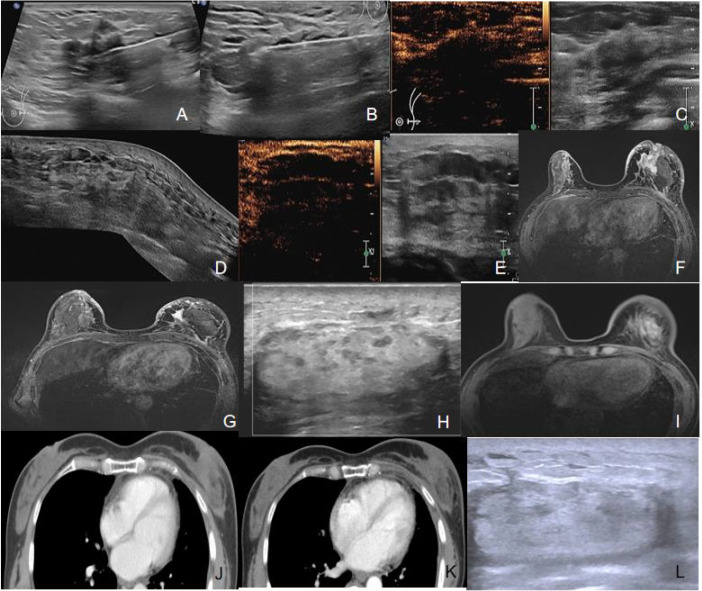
Long-term imaging follow-up after tumor ablation. (A) Pre-ablation biopsy; (B) Intraoperative ablation under ultrasound guidance; (C) Immediate post-ablation contrast-enhanced ultrasound showing no enhancement in the target area; (D) 1-month post-ablation panoramic ultrasound of ablation zone; (E) 1-month post-ablation contrast-enhanced ultrasound demonstrating no enhancement; (F) 1-month post-ablation enhanced MRI showing lesion size reduction compared to baseline; (G) 6-month post-ablation enhanced MRI indicating volume reduction in majority of lesions; (H) 6-month post-ablation ultrasound revealing no discernible mass echo in the region; (I) 1-year post-ablation enhanced MRI demonstrating significant regression of the lesion; (J) 2-year post-ablation enhanced CT showing no significant enhancement to suggest viable tumor; (K) 3-year post-ablation enhanced CT confirming persistent absence of enhancement; (L) 3-year post-ablation ultrasound with no detectable mass-like echo in the region.
